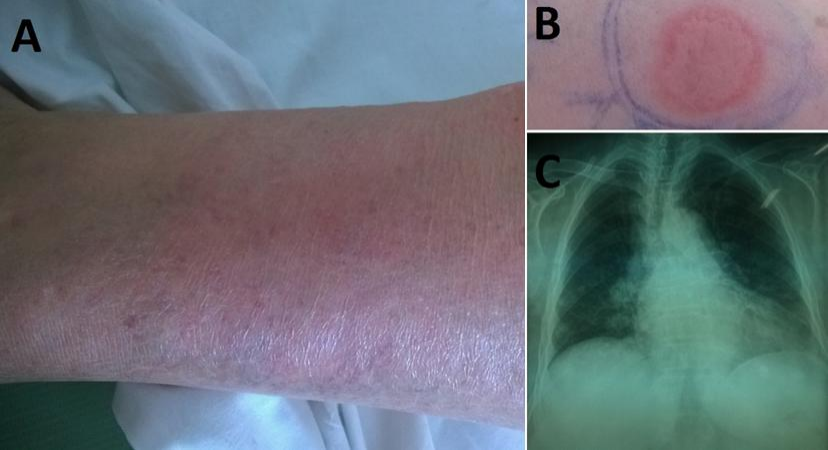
A) Erythema nodosun on the patient's left shin; B) Positive Mantoux test; C) Chest X-Ray demonstrating hilar lymphadenopathy, mainly at the right side
